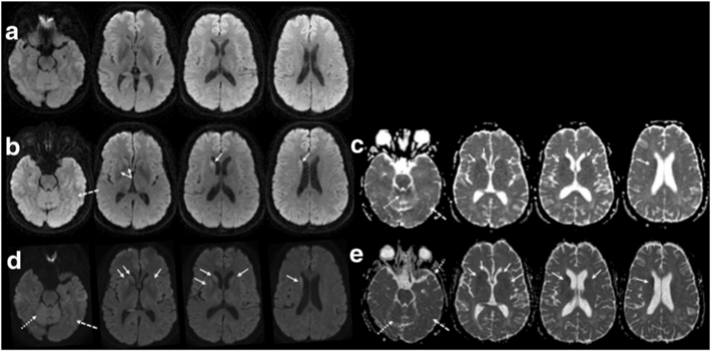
Brain MRIs of index case. a-e: MRI was normal at 4 months without clear evidence of restricted diffusion on DWI sequence (a), but progressed to restricted diffusion in the right caudate head (solid arrows) and more subtle cortical (dashed arrows) and possible superior vermis (dotted arrows) involvement at 10 months (b, DWI; c, ADC map), with progression to bilateral asymmetric caudate and more evident cerebellar vermis involvement at 11.5 months (d, DWI; e, ADC map)
